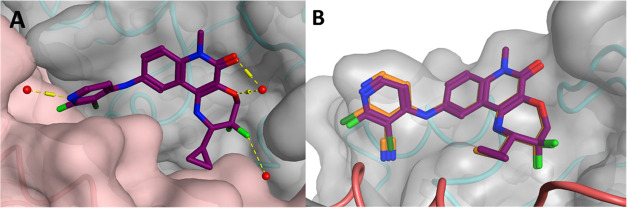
(A) X-ray structure of the BCL6 BTB domain with bound
